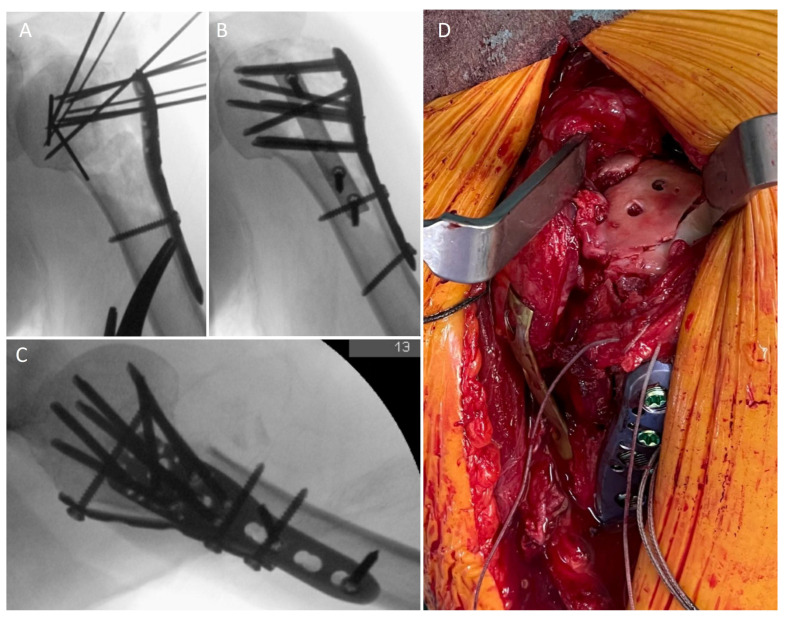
(A–C): Intraoperative X-rays demonstrating the reduction and fixation using the double plating technique of the patient in Figure 1. (D): Intraoperative clinical picture showing an opened-up rotator interval to control the reduction of the articular surface. The one-third tubular plate is placed on the lesser tuberosity.
